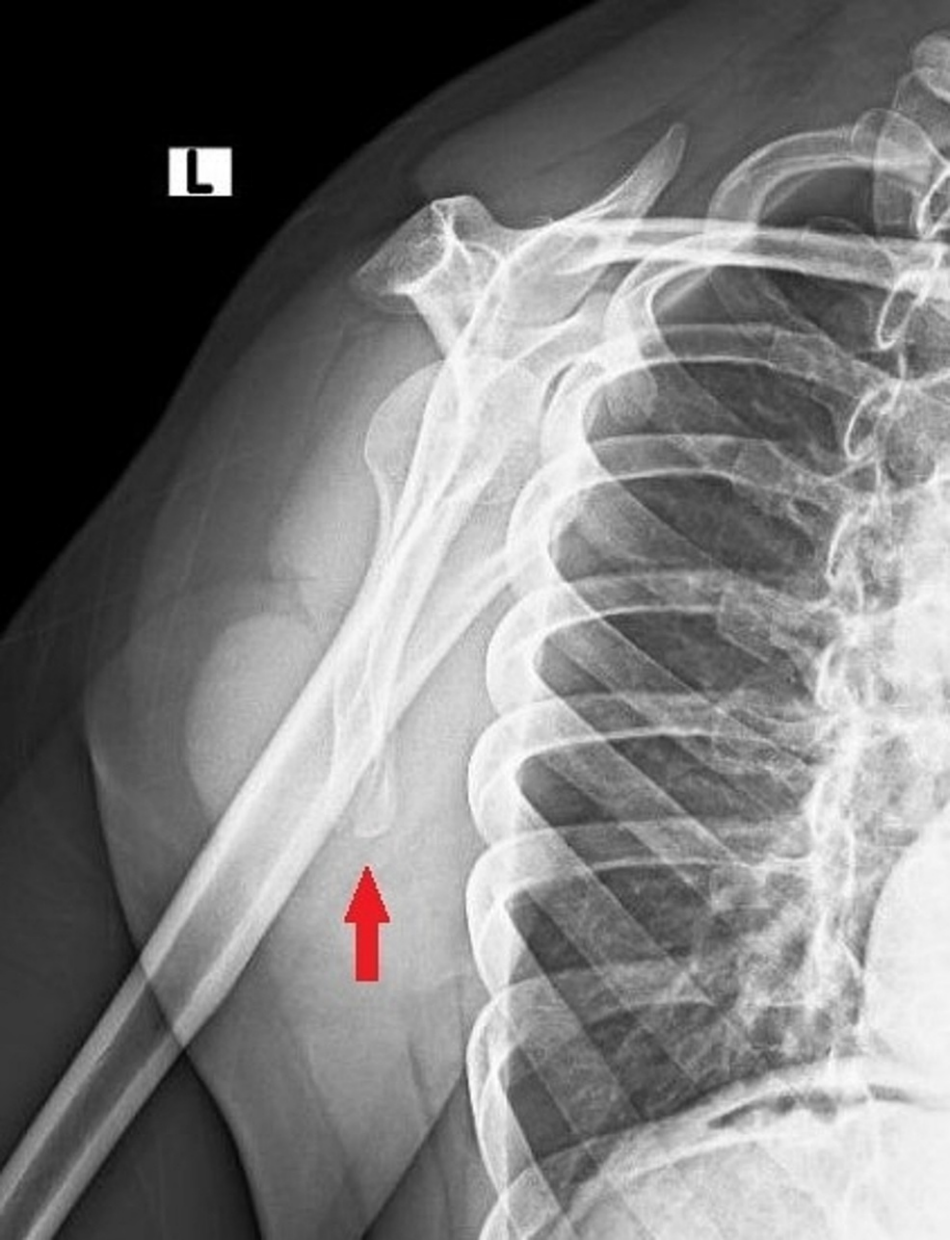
Lateral scapula x-ray revealed the ISAF (red arrow) ISAF: inferior scapula angle fracture
